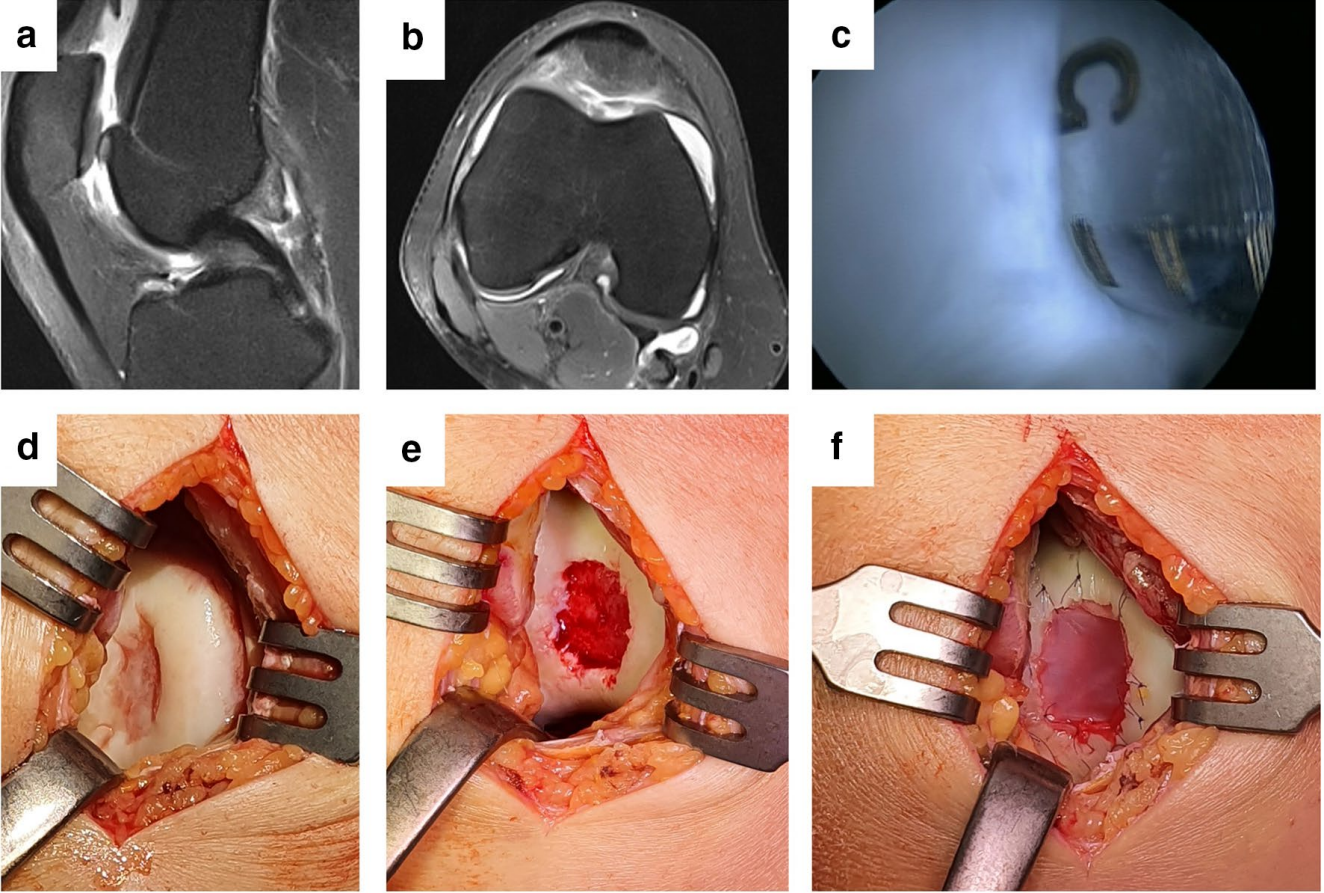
MACT for a large focal degenerative cartilage defect of the trochlea. a, b Preoperative MRI showing the defect; c arthroscopic harvest of an osteochondral plug for chondrocyte culture; d cartilage defect in the trochlea; e defect preparation; f MACT by fixation of the cell-loaded scaffold in the defect with sutures in a mini-open technique
